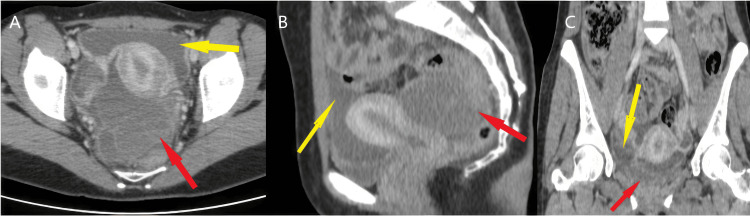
Enhanced CT scan (axial (A), sagittal (B), and coronal (C) views) showing a multi-loculated, retro-uterine, thick-walled, slightly enhancing cyst (red arrow) with intraperitoneal fluid (yellow arrow).
